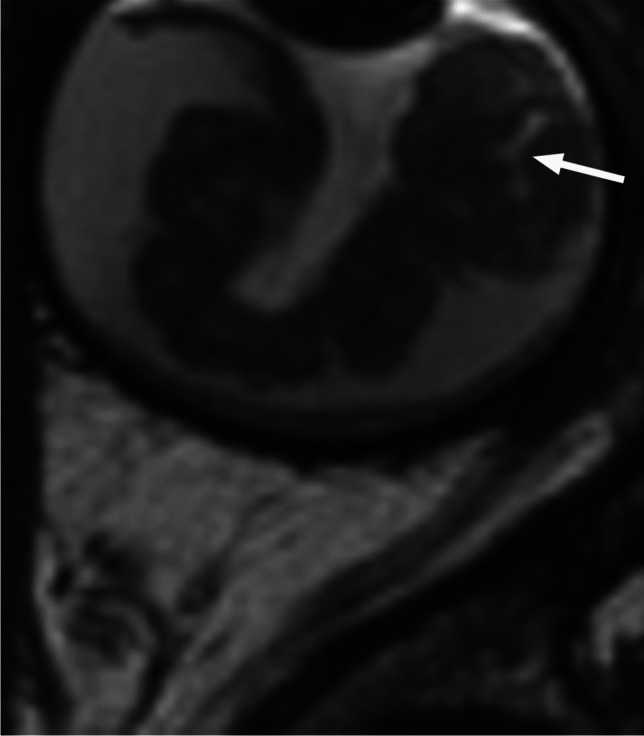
High resolution axial T2-weighted image of the globe reveals retinoblastoma with diffuse, infiltrative growth along the detached retina. There is tumor-retina folding with enclosure of the vitreous (arrow)
